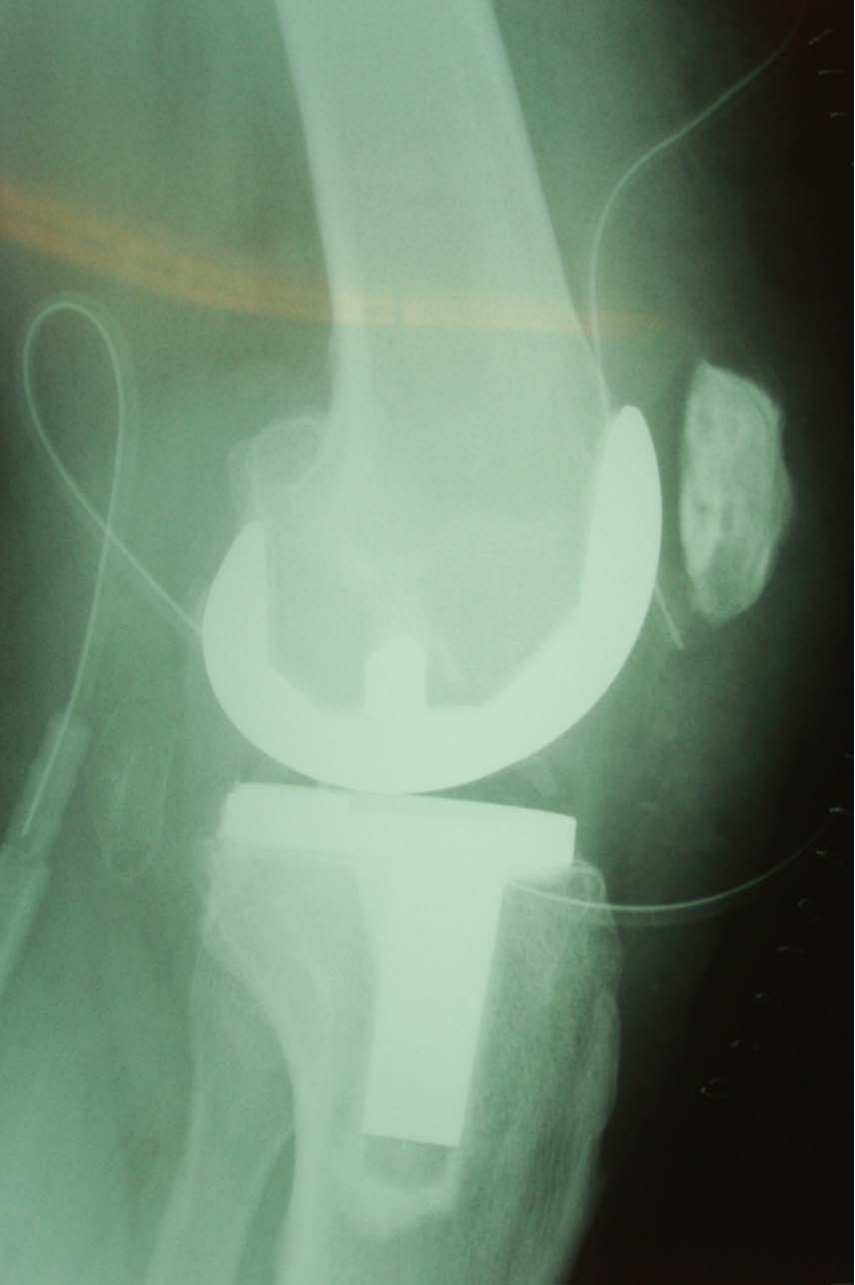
Postoperative sagittal radiograph of the knee after revision of the patellar component.
